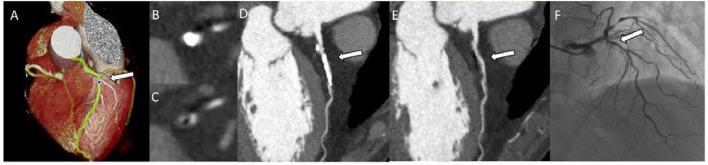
Patient showing stenosis regrading in conventional CCTA and subtraction. VR (A), Axial (B), and CPR (D) images of proximal left anterior descending artery with severe calcification on conventional CCTA. Axial (C) and CPR (E) images for subtraction CCTA. The ICA image (F). The arrow indicates calcified plaque with or without subtraction CCTA, and the same lesion on ICA. CCTA, coronary computed tomography angiography; VR, Volume rendered; CPR, curved planar reconstruction; ICA, invasive coronary angiography.
